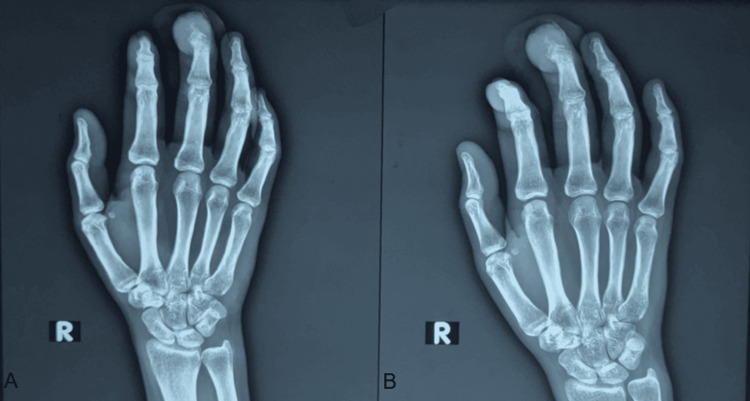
(A, B) Postoperative X-ray
